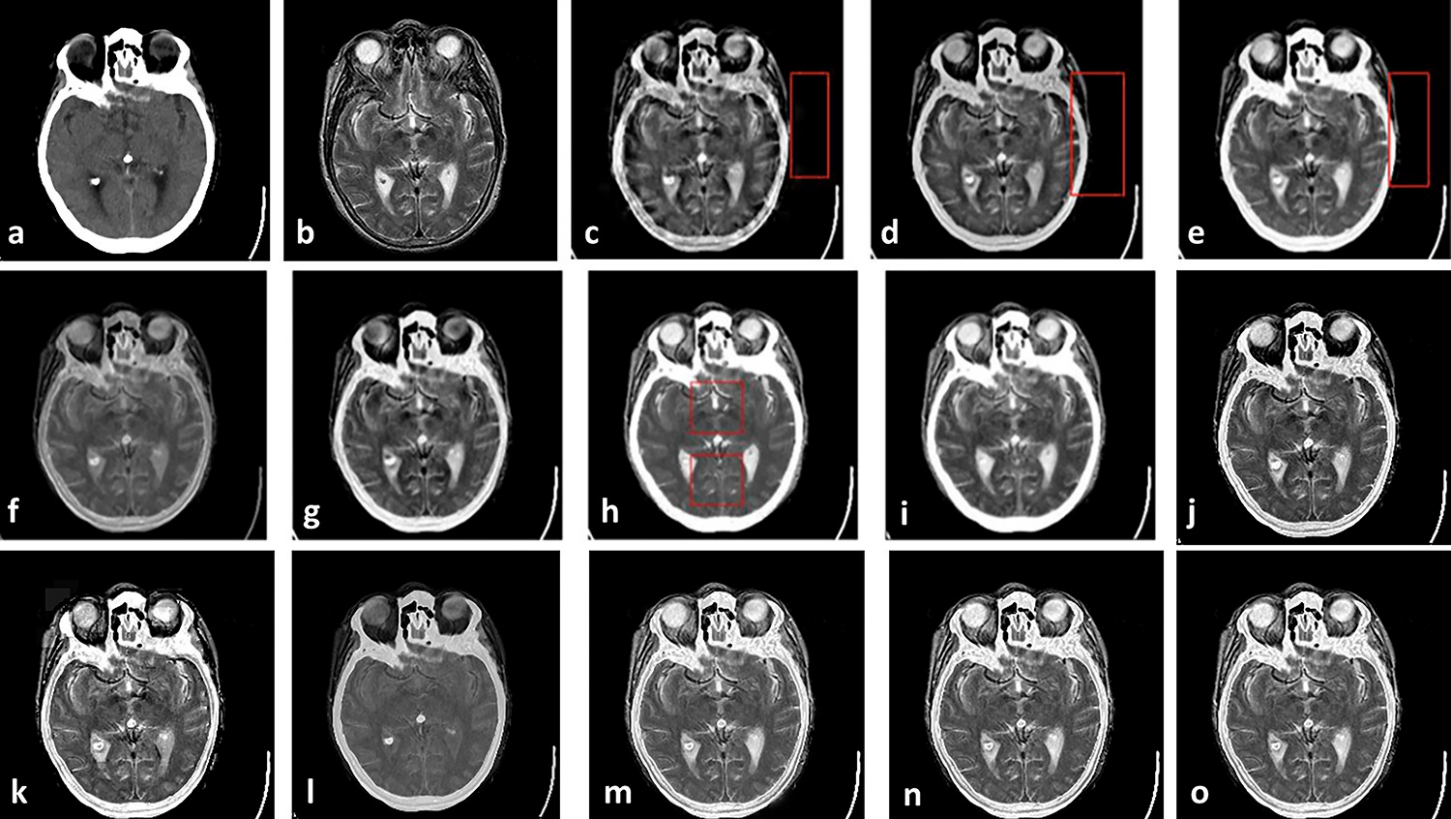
Experiment 2: CT and MR-T2 images of brain with acute stroke, and the fusion results. (a) CT image; (b)MR-T2 image; (c) DWT; (d) NSCT; (e) NSCT-SR; (f) m-PCNN; (g) PCNN-NSCT; (h) SCM-F; (i) SCM-M; (j) Laplacian Pyramid; (k) Morphological Difference Pyramid; (l) PCA; (m) the proposed method with PCA, (n) max, and (o) weighted averaging models, respectively.
